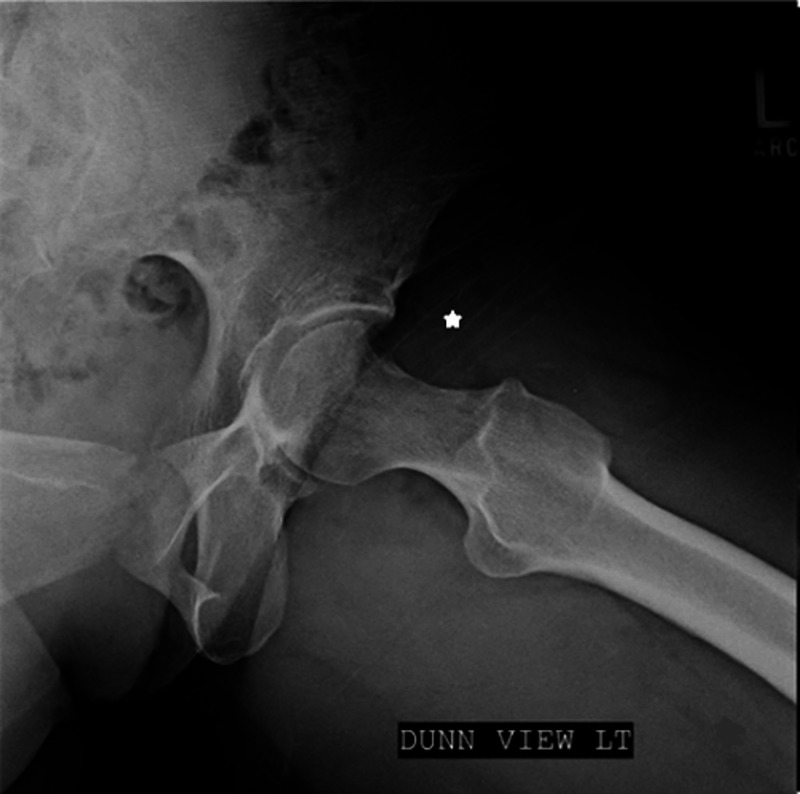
Post-Operative Radiograph Case 1 Modified Dunn radiograph taken 6 months postoperatively. The star indicates that there has been no recurrence of HO.
